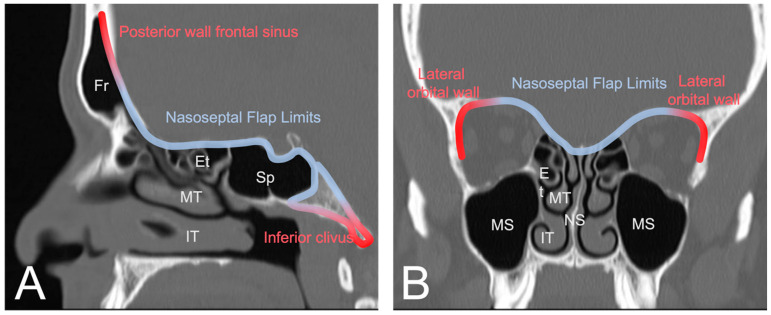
(A) Sagittal view of CT sinus without contrast through frontal sinus outflow tract demonstrating the general reach of the nasoseptal flap (blue). The posterior wall of the frontal sinus and the lower third of the clivus are typically too distal for the conventional nasoseptal flap (red). (B) Coronal view demonstrating lateral reach covering the ethmoidal roof and medial bony orbit (blue) with distal limits at the lateral orbital wall (red). Image from *Radiopaedia*.*org*. Fr: Frontal sinus; Et: Ethmoid air cells; Sp: Sphenoid sinus; MT: middle turbinate; IT: inferior turbinate; MS: maxillary sinus; NS: nasal septum.
